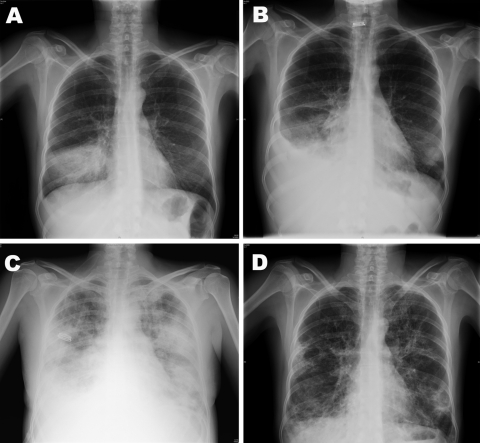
Chest radiographs of 37-year-old, HIV-positive woman with severe pandemic (H1N1) 2009 virus infection, 2009. A) June 24, alveolar infiltrate in the right lower lobe. B) July 3, minimal pleural effusion, alveolar infiltrate on right lower lobe, and possibly left lower lobe infiltrate. C) July 6, bilateral alveolo-intertitial infiltrates. D) July 29, bilateral peribroncovascular thickening with fibro-cicatricial changes; conserved lung volumes.
